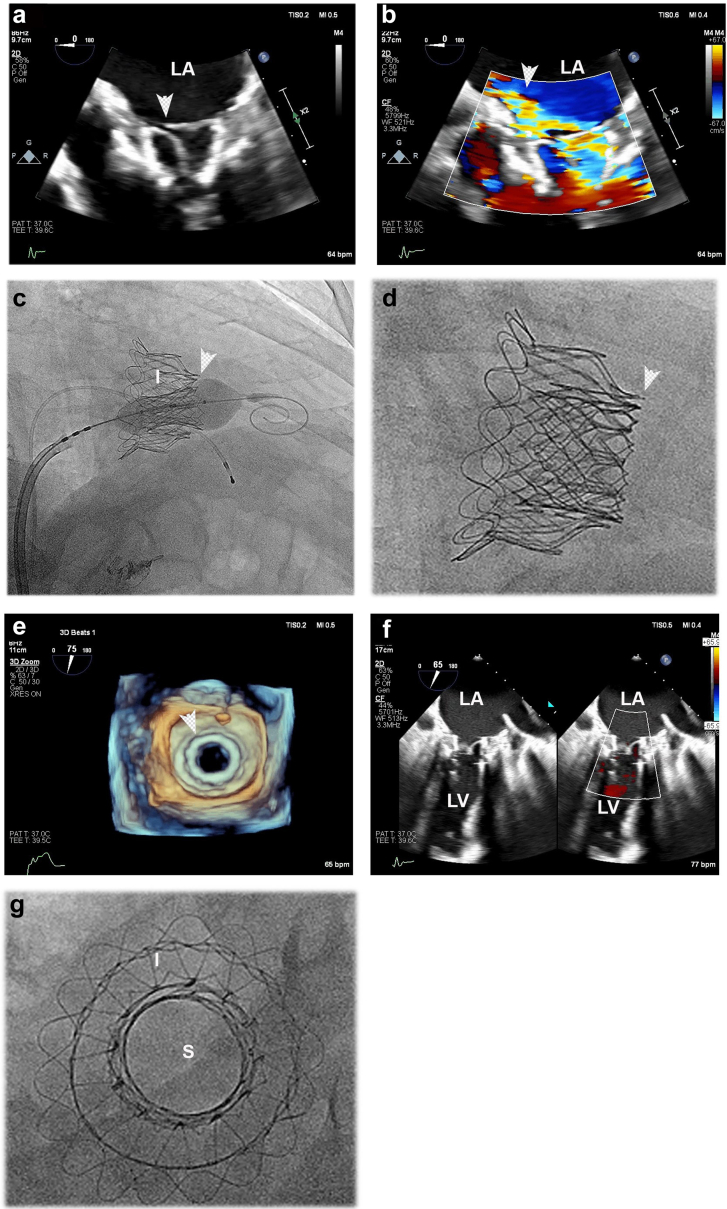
Mitral valve-in-valve therapy for an Intrepid prosthesis with chronic degeneration. (a) Transesophageal echocardiography (TEE) shows a flail segment (arrowhead) in a patient who had Intrepid implantation 5.5 years previously. (b) Severe mitral regurgitation (MR) is present (arrowhead). (c) and (d) A 26 mm Sapien valve is placed with matching of the outflow frames of the 2 prostheses (arrowheads). (e) The Sapien (arrowhead) is fully expanded. (f) Complete relief of MR occurs. (g) En face view of the final valve-in-valve implantation. Abbreviations: I, Intrepid prosthesis; LA, left atrium; LV, left ventricle; S, Sapien prosthesis.
